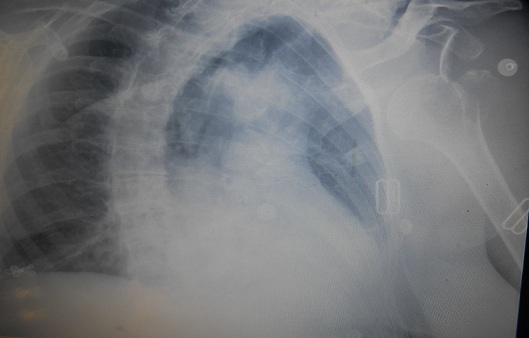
La radiographie de l’épaule objectivant une luxation antéro-interne sous coracoïdienne avec arrachement de trochiter
